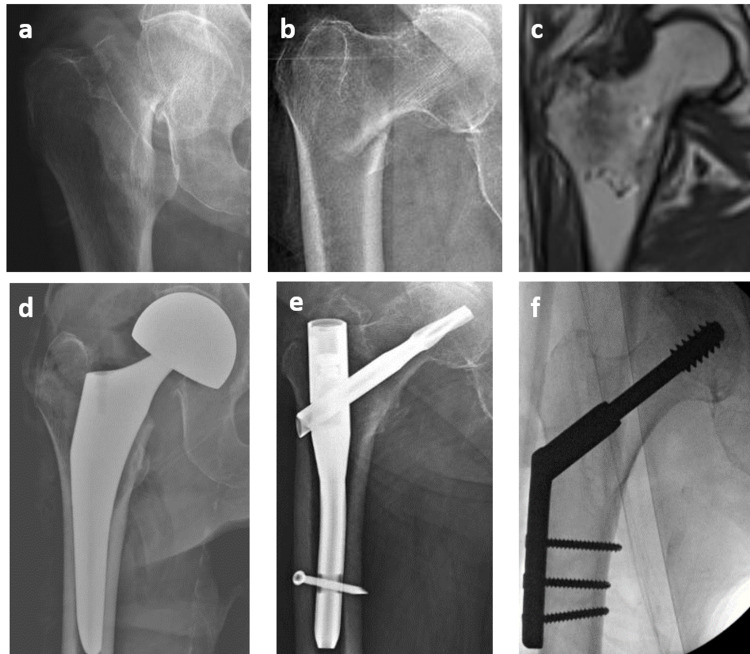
Radiographs of various peritrochanteric proximal femur fractures and their commonly performed surgical treatment methods 2-a and 2-b are radiographs of a displaced femoral neck fracture and a displaced intertrochanteric femur fracture, respectively. 2-c is an MRI of a nondisplaced intertrochanteric femur fracture. 2-d through 2-f are postoperative radiographs of the corresponding fractures above, demonstrating a hemiarthroplasty, a cephalomedullary nail, and a sliding hip screw, respectively.
